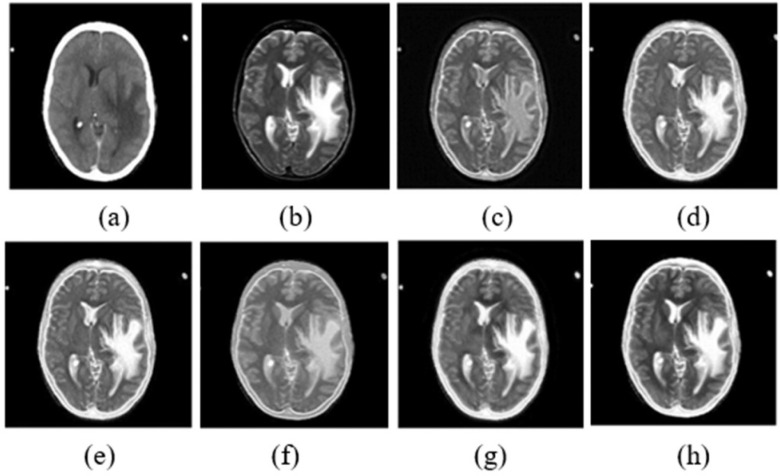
MRI-CT fusion (a) CT input, (b) MRI input, (c) MCA-CS [14], (d) LTEM [15], (e) DB-CNN [17], (f) GF-SDL [18], (g) LDNSD [19], (h) Proposed fused outcome.
